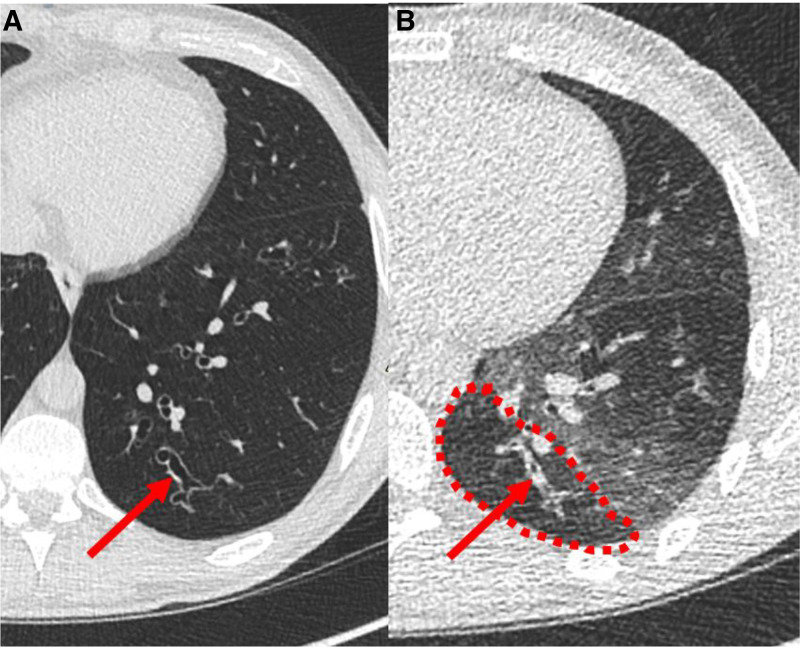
Axial computed tomography (CT) with inspiratory image left (*A*) and expiratory image right (*B*), subsegmental posterior-basal left lower lobe. The airway pointed out with the red arrow is patent, but ectatic on the inspiratory image, but collapses on the forced expiratory image. The adjacent low attenuation (inside red dashed line) is generally interpreted as “small airways disease” or “trapped air,” but the nonphysiologic larger airways collapse could contribute to this appearance. This participant had a percent predicted forced expiratory volume in 1 second (ppFEV_1_) of 83% and *Z*-score of −1.36. The location of the collapsed airway is at the 6th generation, which is still within the proximal airways.
